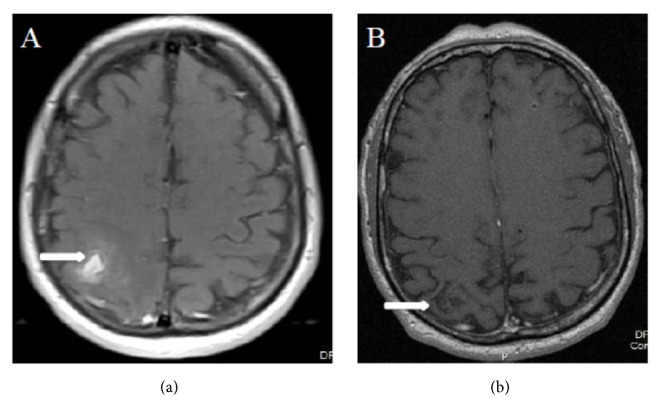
MRI brain*. (a) An axial, postcontrast-enhanced, fat-saturated T1-weighted image of the brain shows a 2.0 x 1.0 cm right parietal mass with ill-defined area of enhancement. (b) An axial, contrast enhanced, fat-saturated T1-weighted image of the brain, 3 weeks after therapy with cabozantinib, shows resolution of the prior mass and surrounding enhancement.
